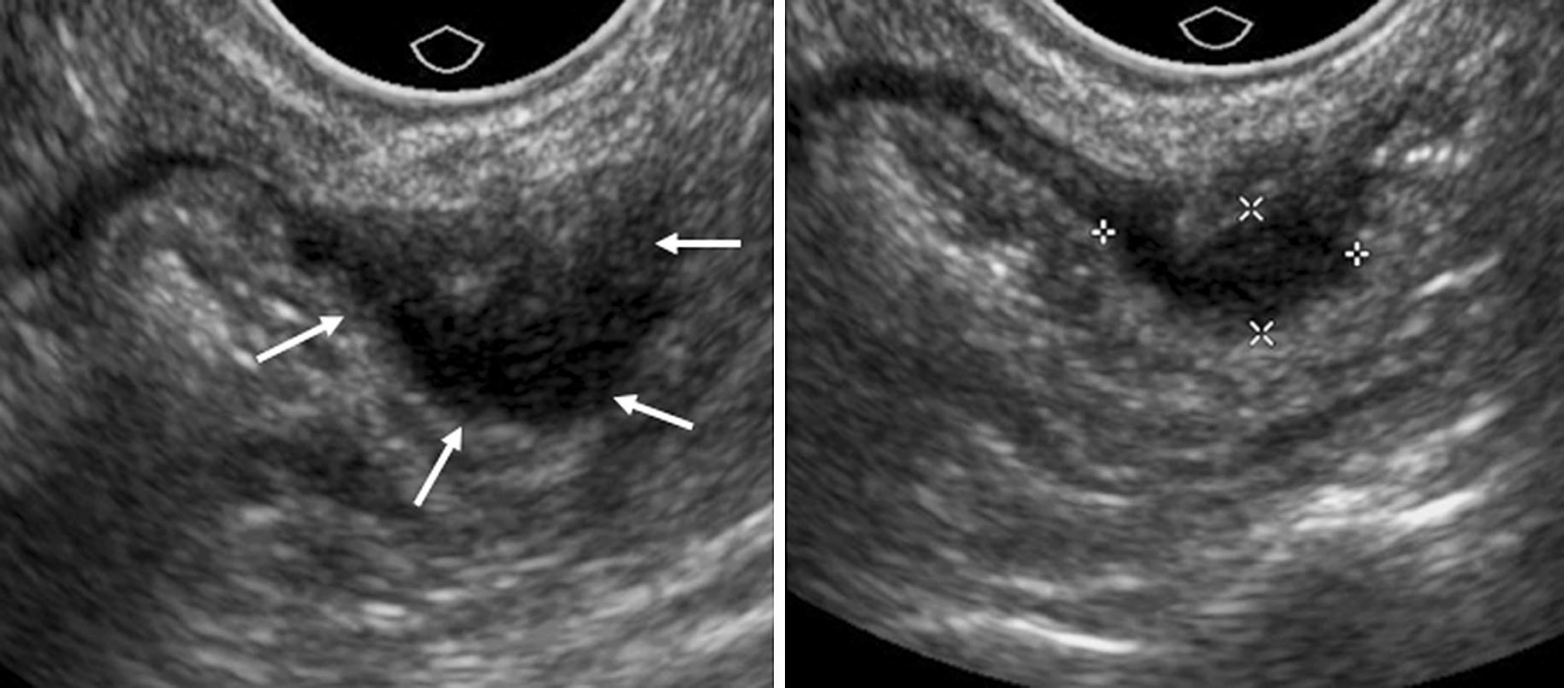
Expert transvaginal ultrasound (ETVUS) images showing endometriosis involving the anterior rectum, appearing as a solid, non-compressible, hypoechoic implant (*white arrows*, electronic calipers). Unlike routine transvaginal ultrasound, ETVU assesses the rectum and sigmoid for improved sensitivity.
